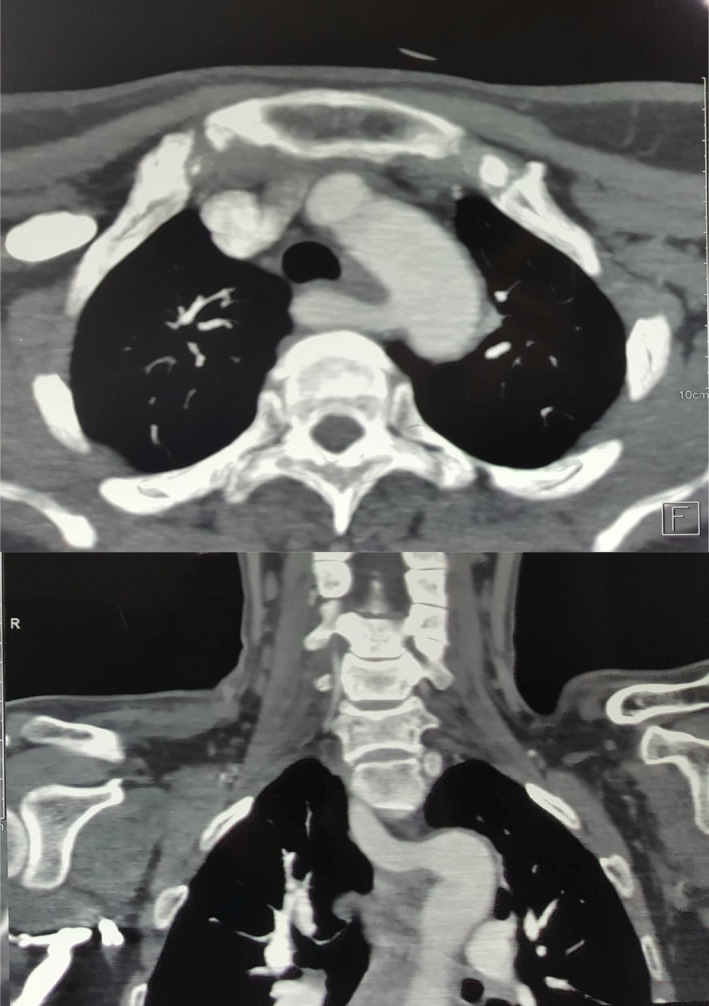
Axial and coronal CT scan, arterial phase, showing an ectopic vessel originating from the aortic arch and crossing the midline between the esophagus and the vertebral column to form the right subclavian artery (arteria lusoria)
